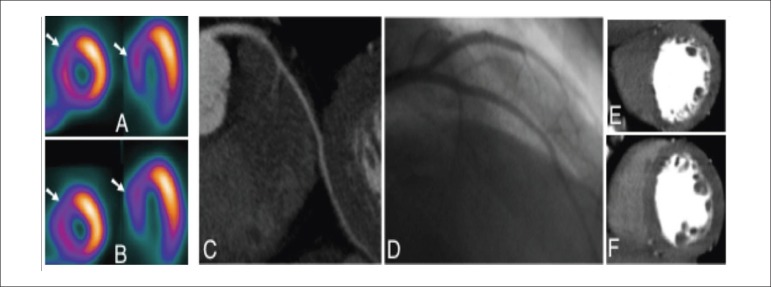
Patient number 30, a SPECT false-positive caused by LBBB. (A) Stress and (B) rest
